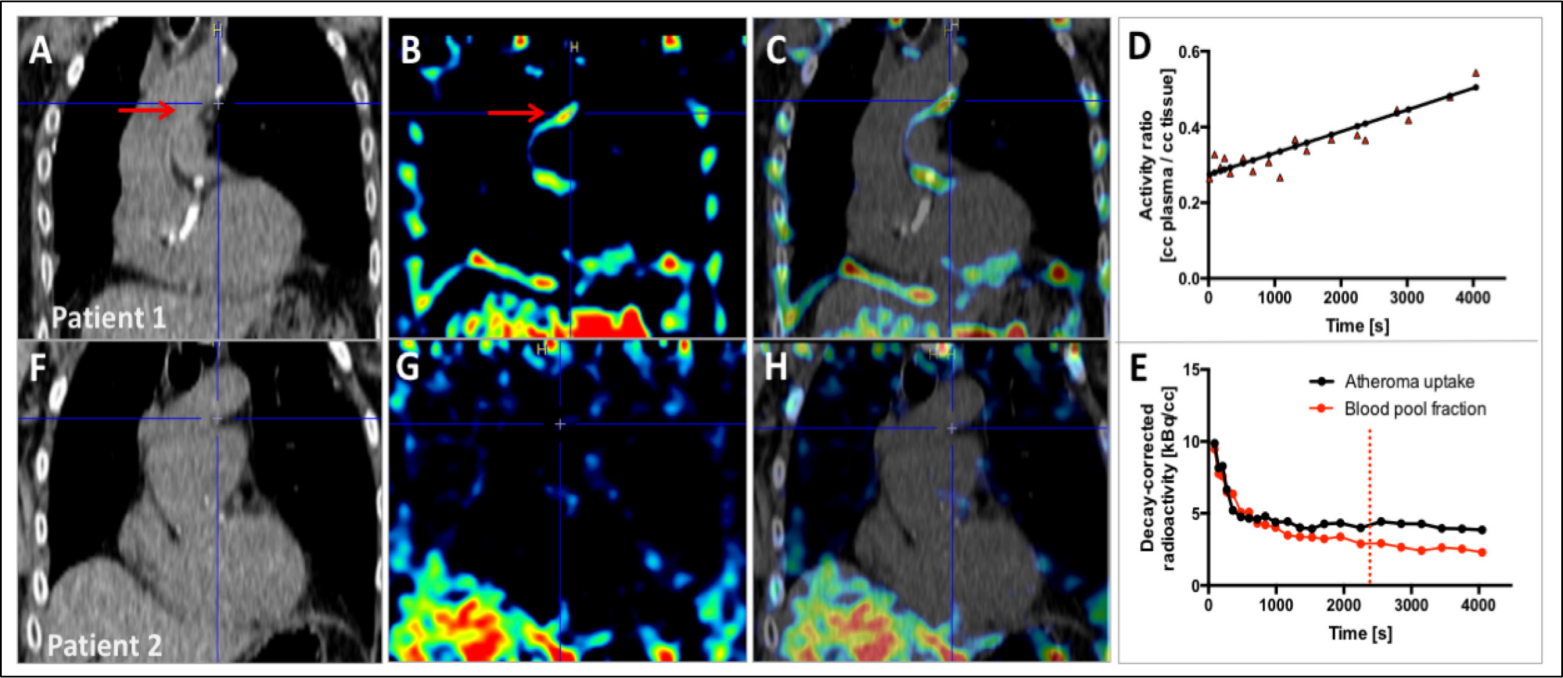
Kinetic analysis of aortic 18F-fluciclatide uptake. Sagittal views of the thorax following kinetic analysis in two participants with (patient 1: A–E) and without (patient 2: F–H) aortic arch 18F-fluciclatide uptake. CT images confirm presence (A) or absence (F) of aortic arch calcification as a marker of established atheroma. Patlak slope (Ki) parametric images (B and G) identify focal uptake within the aortic arch in the region of atheroma (red arrow) localising to the vessel wall on the fused Patlak and CT images (C and H). Patlak modelling (D) confirms irreversible integrin binding within the region of aortic arch calcification (red arrow). Time activity curves (TAC) within the same region (E) show a persistently high blood pool fraction, but uptake within atheroma that exceeds the blood pool fraction beyond 40 min (dashed line).
